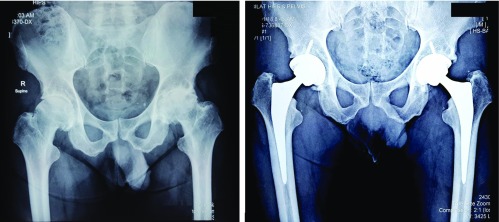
Pre-operative AP X-Ray pelvis with both hip joints (Case 1) showing grade IV OA both hip joints and one year follow-up post-operative X-ray after bilateral uncemented bikini hip replacement showing well aligned acetabular and femoral prosthesis in situ. (Case 1). During surgery the stem was prepared first followed by the cup. Prosthesis details: Acetabular shell: R3 three hole HA acetabular shell (Smith and Nephew Memphis Tennessee). Acetabular liner: R3 XLPE (Smith and Nephew). PolarStem femoral stem (uncemented − Smith and Nephew AG, Baar, Switzerland), femoral head: oxinium femoral head (Smith and Nephew Memphis Tennessee). The total operative time was 2 h and 15 min.
